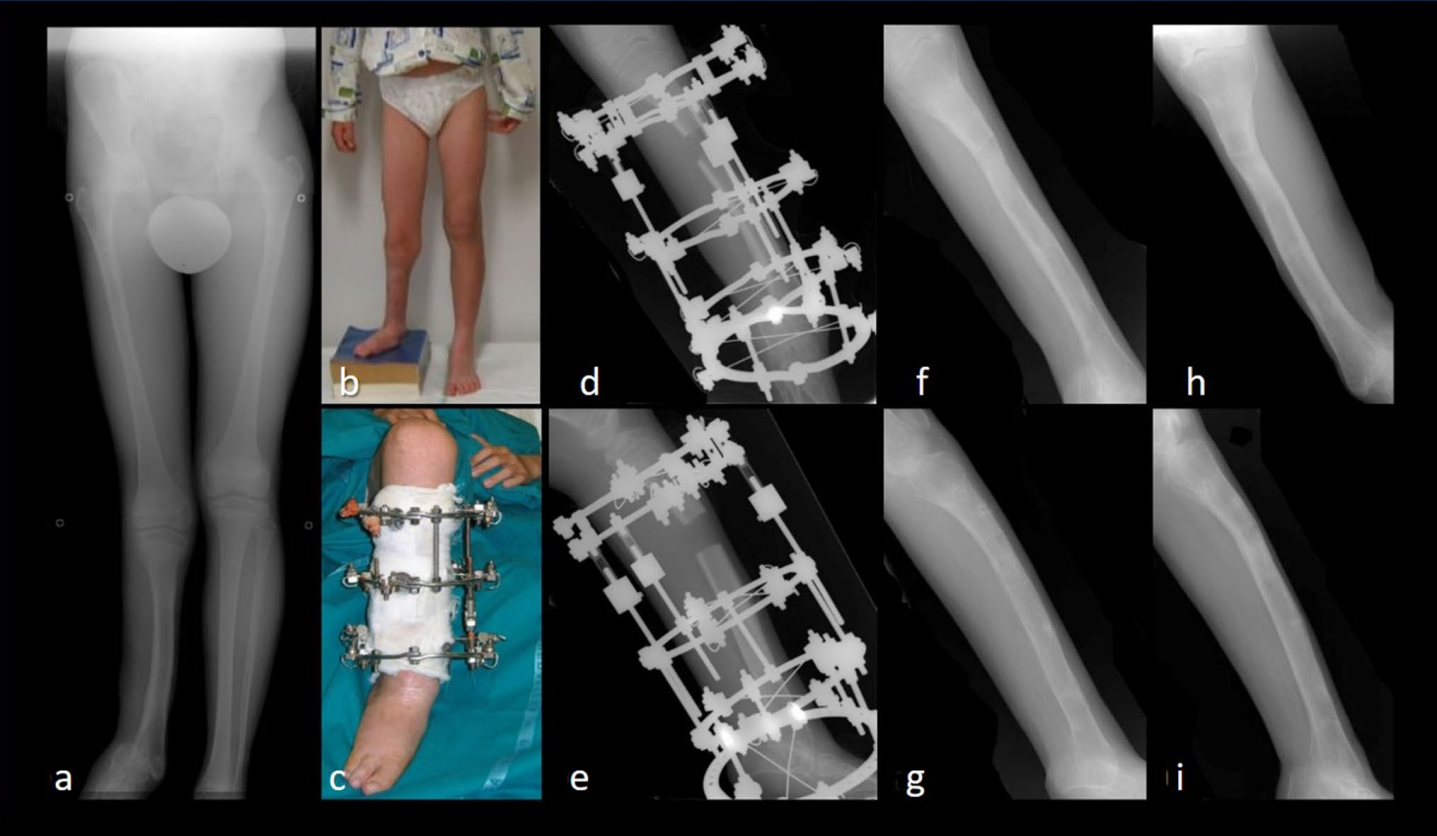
A 15-year-old patient affected by longitudinal lateral hemimelia of his right leg with complete absence of the fibula: preoperative AP X-ray (a) and clinical (b) images, showing 7-cm hypometria on the right lower limb. Ilizarov bone transport: clinical image (c) and X-rays on AP (d) and LL (e) during treatment. Radiographic results on AP and LL X-ray views after removal of the external fixator one year later (f, g) and at final follow-up of 18 months (h, i)
